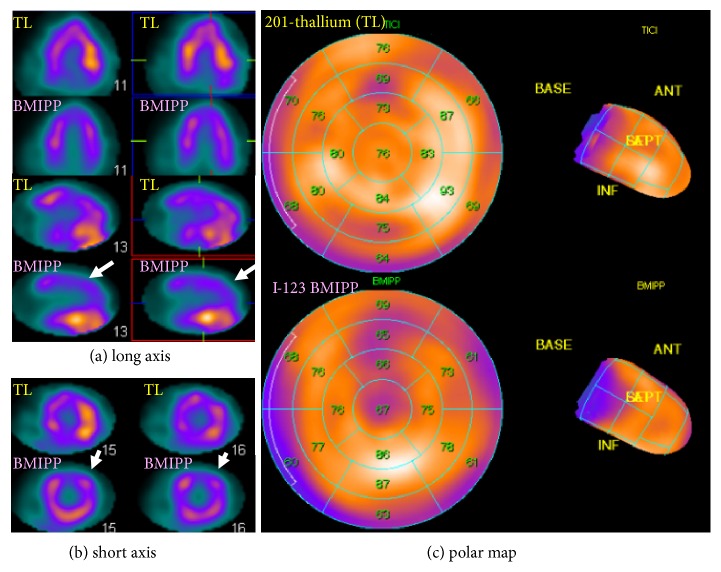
Thallium-201 and iodine-123 β-methyl-p-iodophenylpentadecanoic acid dual-tracer myocardial scintigraphy 3 weeks after admission*. Slight patchy deficit was found for myocardial thallium uptake in the anterolateral wall and further decreased uptake of BMIPP, which was consistent with a mild perfusion-metabolism mismatch (white arrows). (a) Long-axis 2D view. (b) Short axis 2D view. (c) Polar map. 2D, two-dimensional; ANT, anterior; INF, inferior; TL, thallium.
